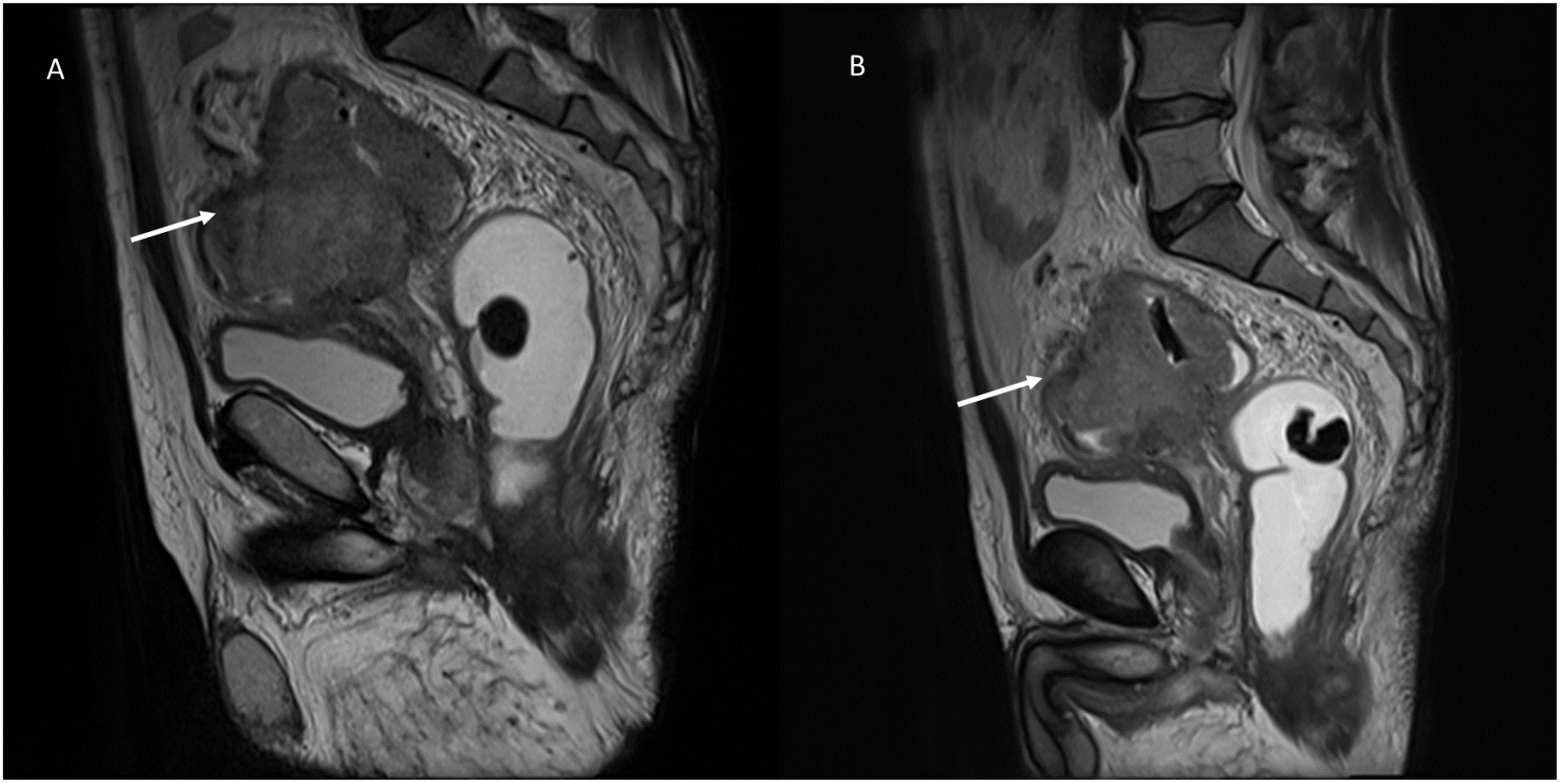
MRI evaluation of chemotherapy response in a patient with recto-sigmoid cancer. Sagittal T2-weighted MRI sequences of a patient, obtained in the pre-treatment (A) and post-treatment phase (B) after chemotherapy. (A) The pre-treatment image demonstrates a large, irregularly marginated mass (white arrow) with intermediate-to-hyperintense signal intensity relative to the surrounding soft tissues. The tumor partially encroaches on the adjacent structures. (B) The post-treatment image acquired after chemotherapy reveals a significant reduction in tumor volume (white arrow), indicating a favorable treatment response. Despite the size reduction, the lesion maintains similar T2-weighted signal intensity compared to the baseline examination. This figure highlights the importance of volumetric assessment in chemotherapy response evaluation, emphasizing that tumor shrinkage is a common indicator of therapeutic efficacy. From Vanzulli and Albano, (42).
